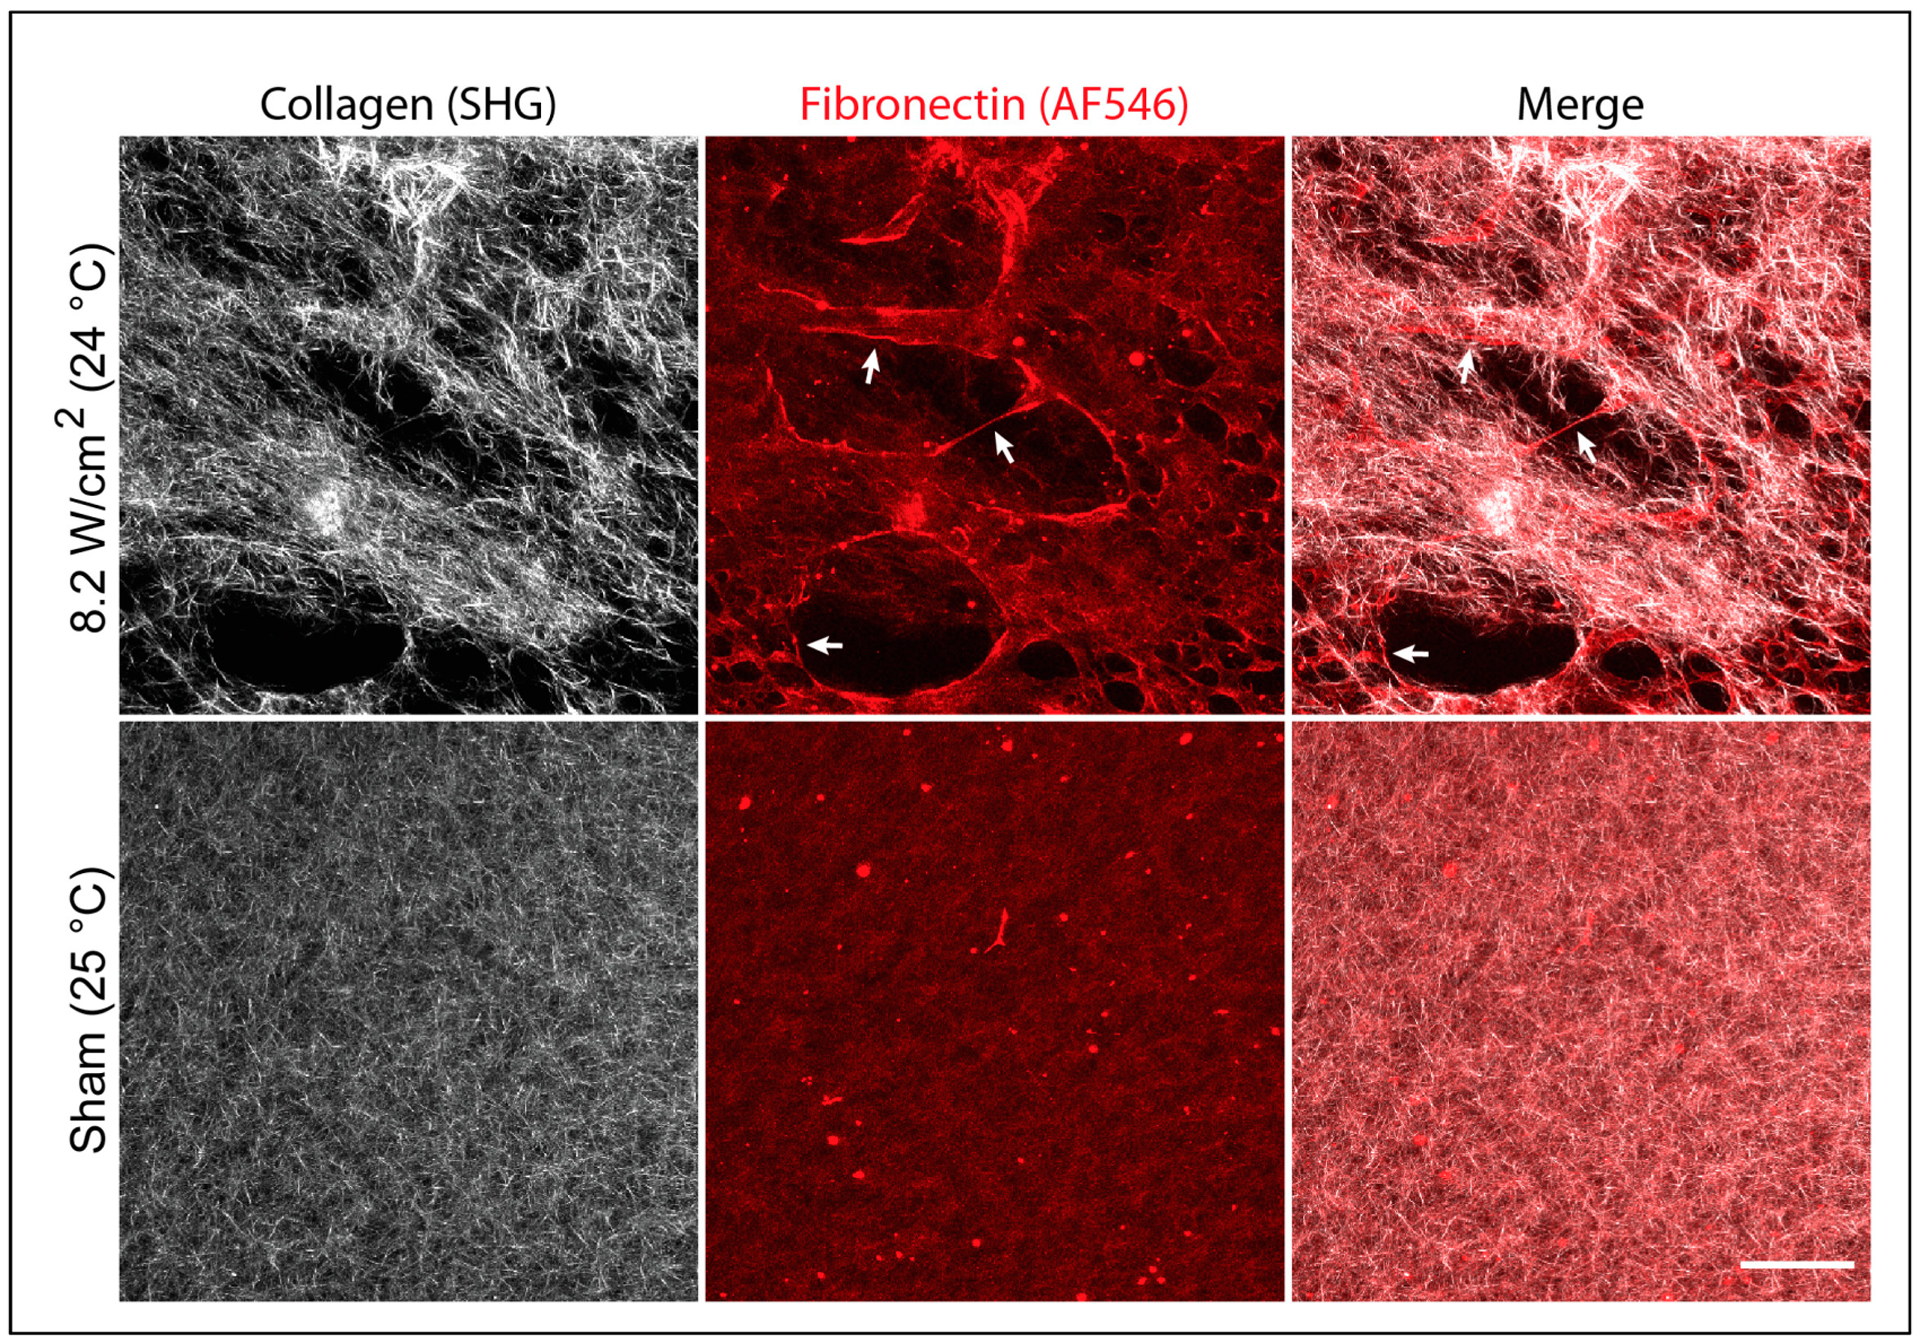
Mechanical effects of ultrasound trigger fibronectin fibril formation under permissive temperature conditions. Neutralized solutions of type I collagen (2 mg/mL) and fibronectin (25 μg/mL, 10% AF546-labeled) were co-exposed to either 8.8-MHz ultrasound at an intensity of 8.2 W/cm^2^ in an 18 °C water bath or sham-exposed in a 25 °C water bath such that the peak temperature achieved within each sample during exposure was 24–25 °C. Unbound fibronectin was removed by media exchange prior to fixation and multiphoton imaging of collagen (white) and fibronectin (red) and fiber structures. Maximum intensity z-projection images through a depth of 70 μm (5-μm z-steps) at the gel surface were assembled using FIJI software. Representative images from 1 of 3 samples per condition fabricated on independent days. Scale bar = 100 μm. Arrows indicate the presence of fibronectin fibrils.
